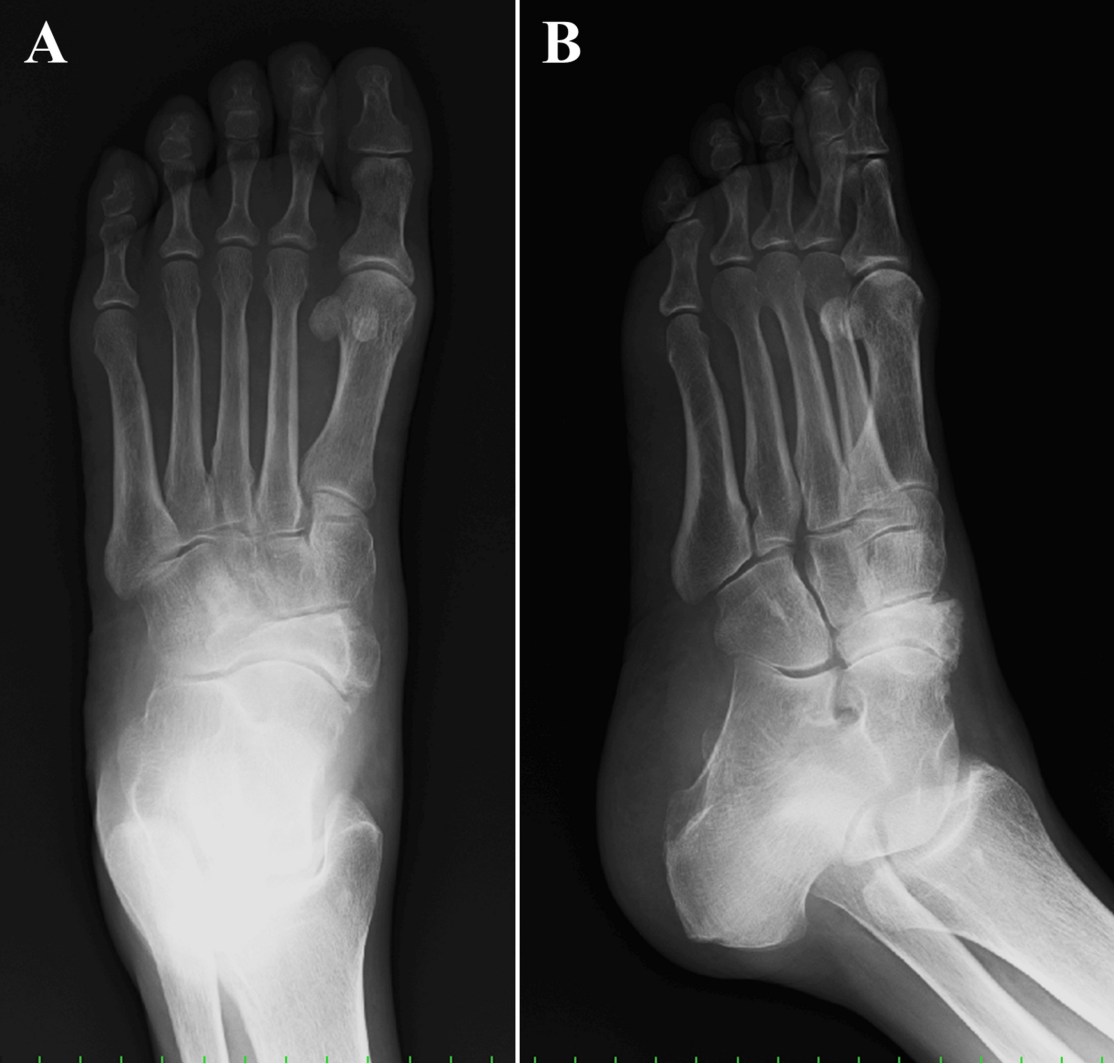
Preoperative plain radiographic findings. (A) Anteroposterior view and (B) internal oblique view.
